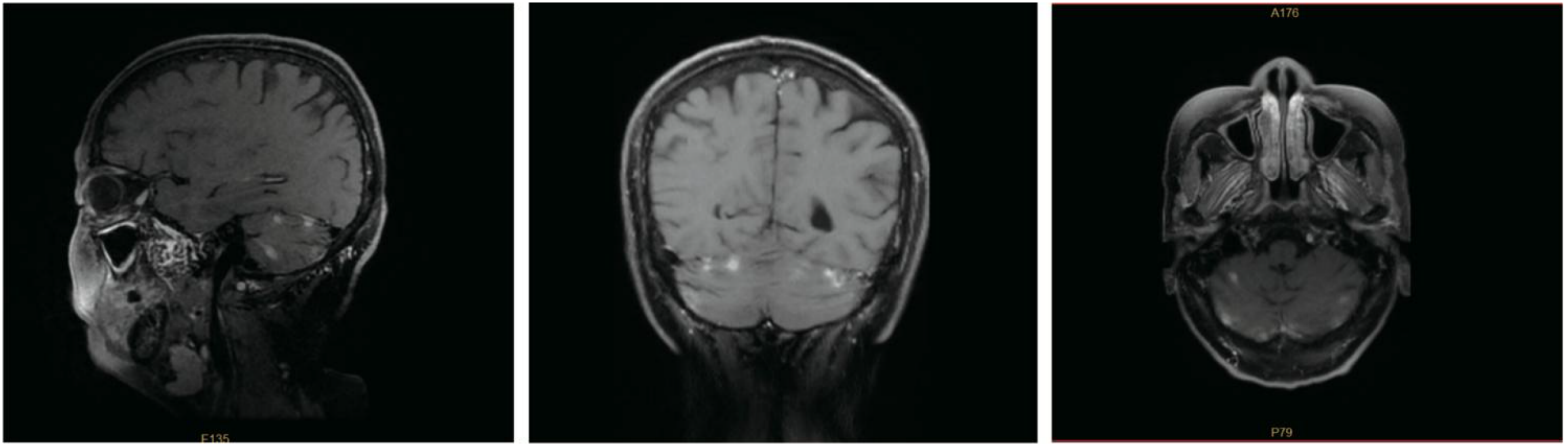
Contrast-enhanced brain MRI on 06 Apr 2025 showing multifocal enhancing lesions in both cerebellar hemispheres, consistent with brain metastases.
